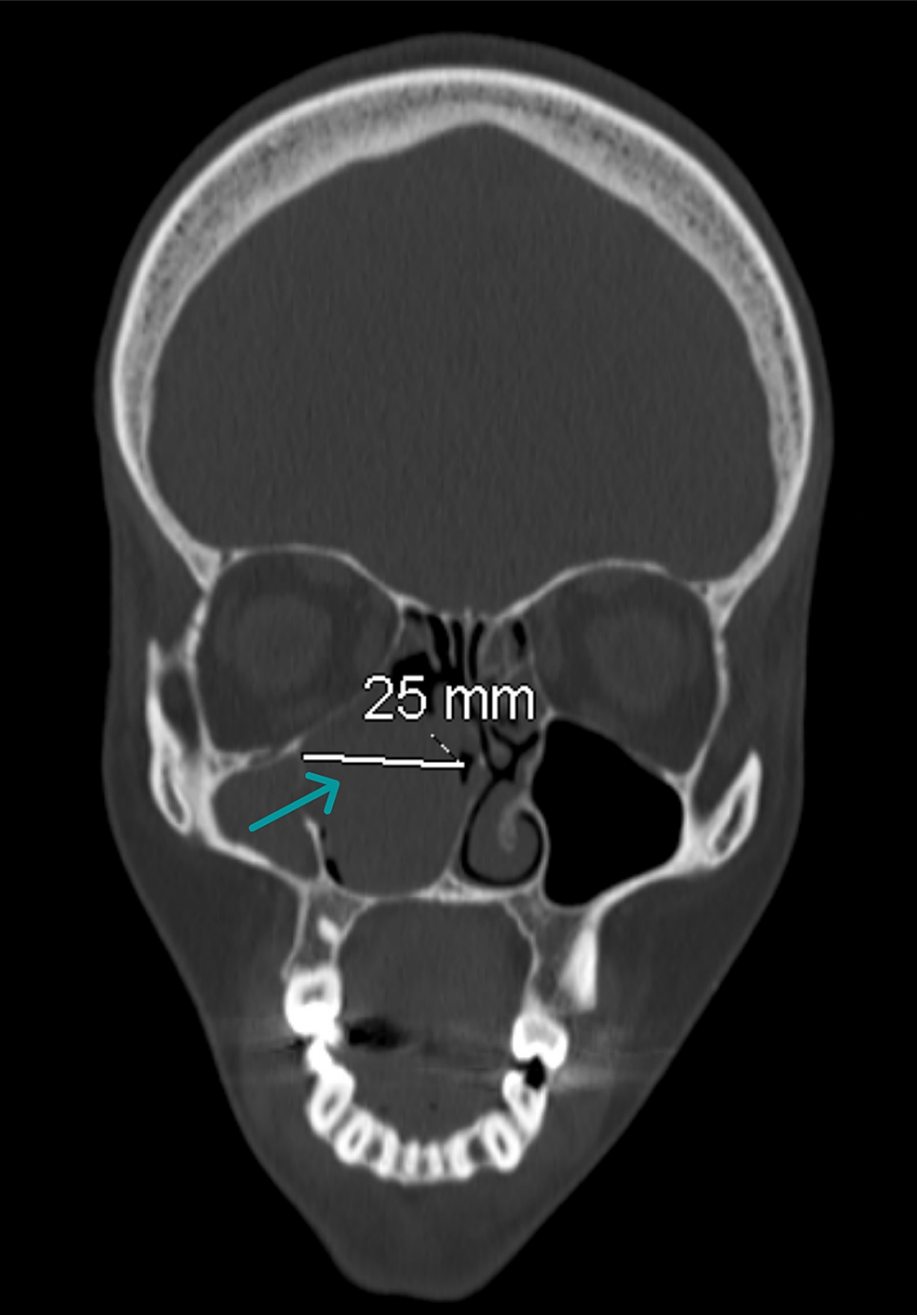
Preoperative CT of the paranasal sinus The coronal view shows the measurement of a concha bullosa mucocele that fills the right nasal cavity and an opposite nasal septum deviation.
